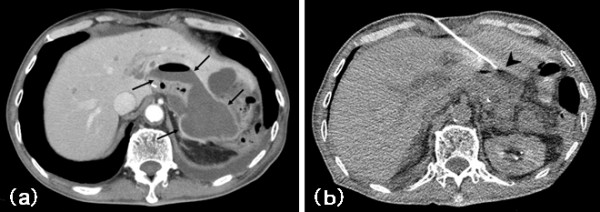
A 76-year-old man was operated for the gastric-cancer 7 days ago. Enhanced CT shows abscess (arrows) in the operated area (Fig. 4a). GNW was accessed to abscess (arrowhead) (Fig. 4b). We used this accessed route for drainage. The lesion was located 9 cm from the needle entry site.
